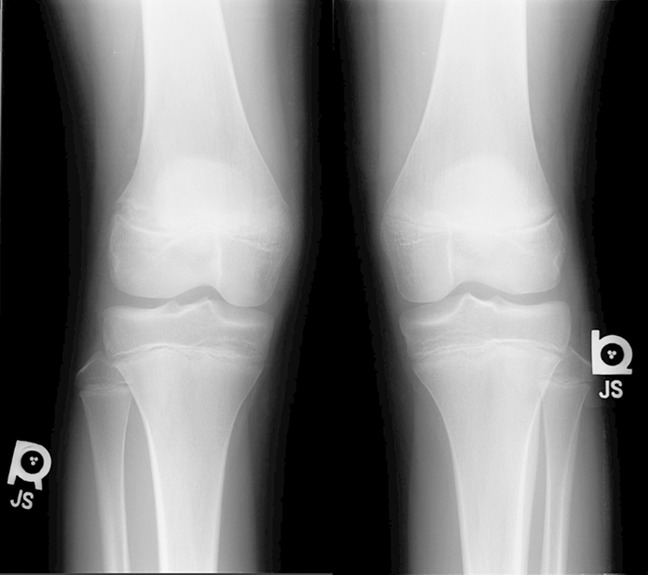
Radiographs of an adolescent boy with atraumatic lateral distal femoral physeal arrest. The right side (affected side) demonstrates physeal widening, while the left side (nonaffected side) demonstrates a normal appearing physis.
